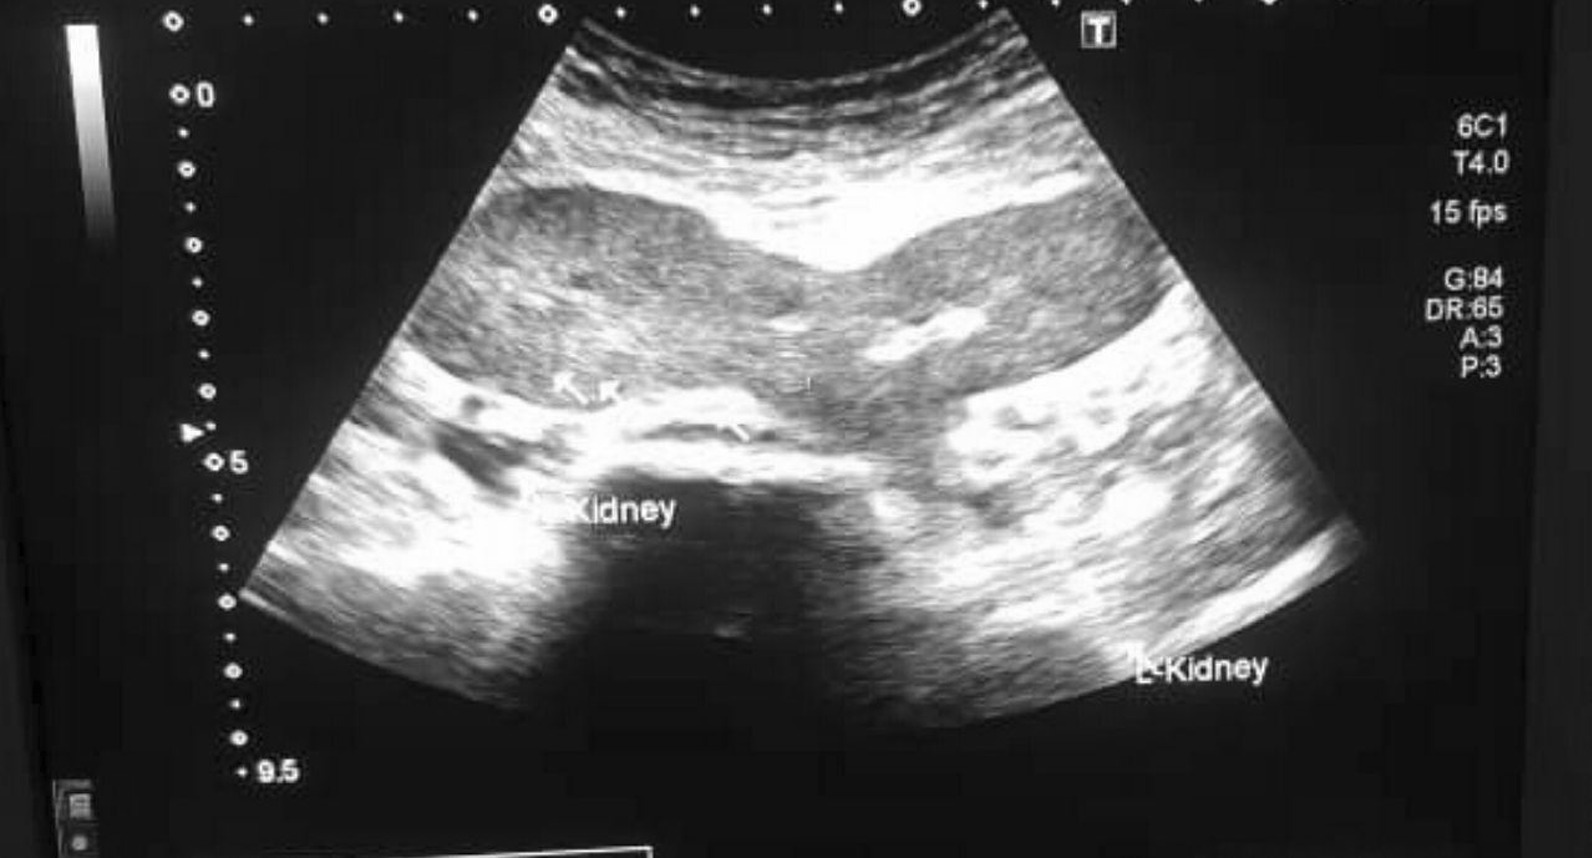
Abdominopelvic ultrasound scan showed ectopic kidneys at the hemi-pelvis, fused in their upper poles, normal size and texture of the kidneys with normal corticomedullary differentiation, no stones or obstructive changes
